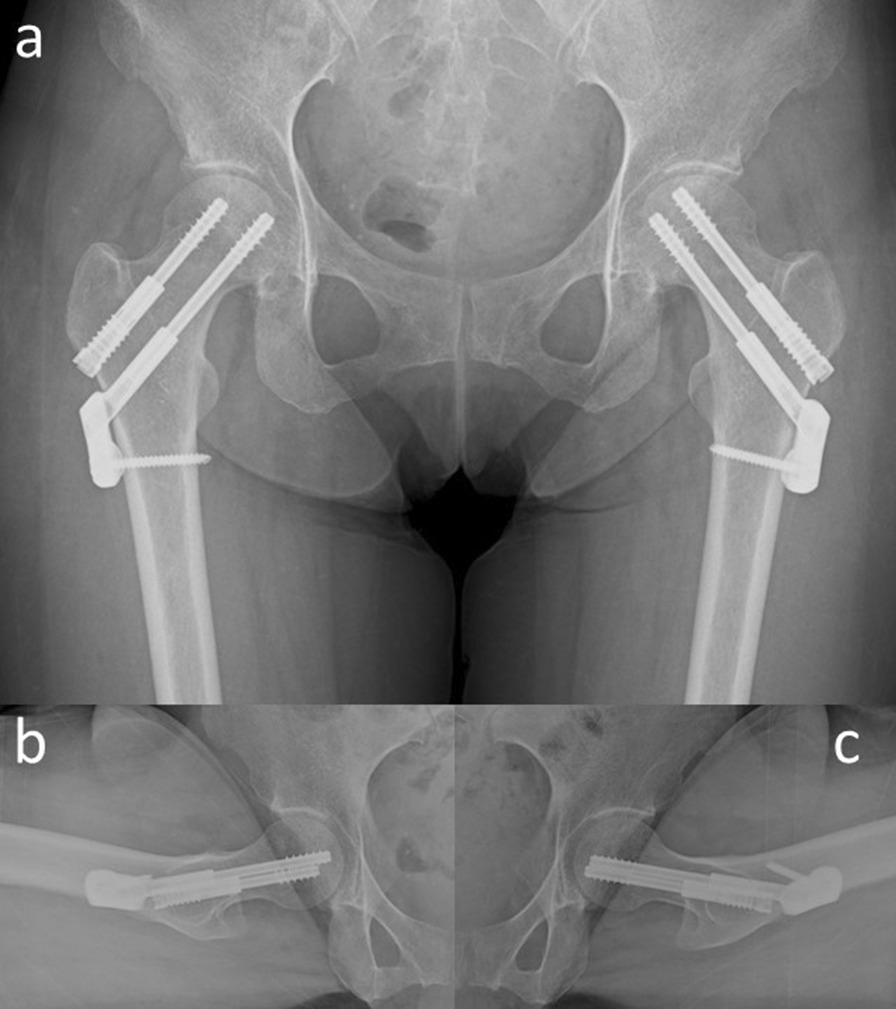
Plain radiographs a–c immediately after the surgery. Anteroposterior a and lateral (Sugioka) (b right hip joint; c left hip joint) views demonstrate the inserted sliding screws without fracture displacement
